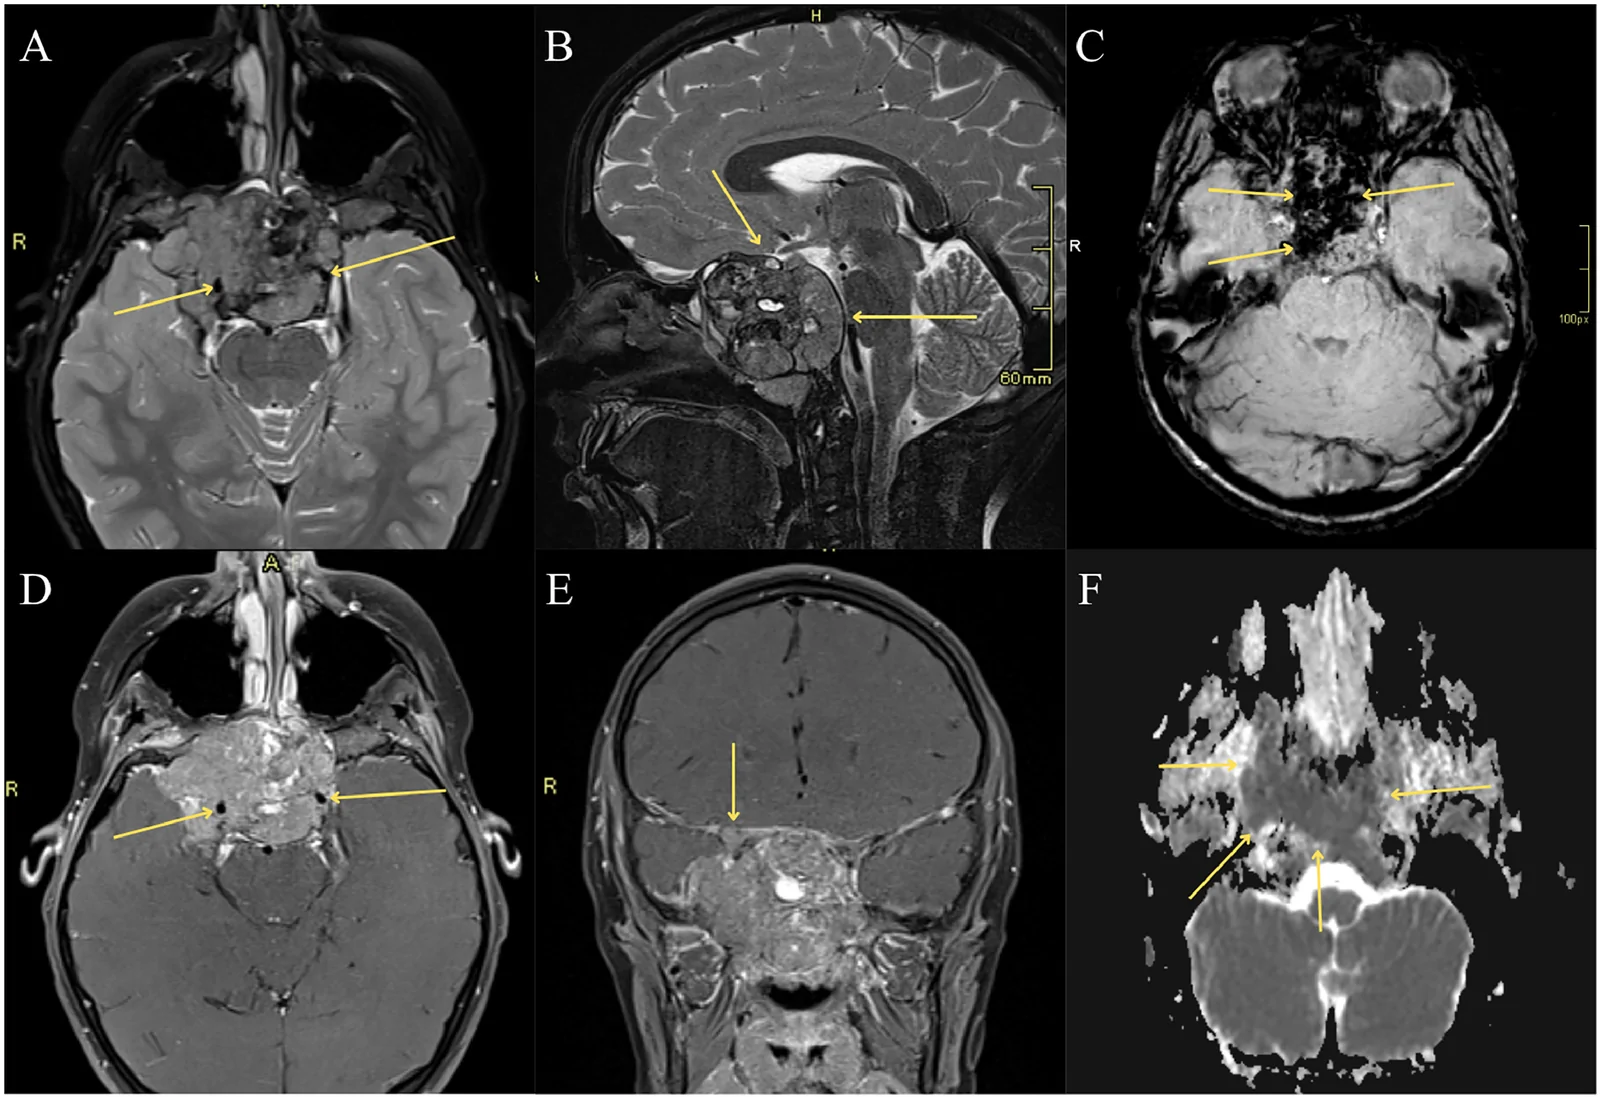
Multiplanar MRI of SMARCB1-deficient sinonasal carcinoma in an 8-year-old patient. (A) Axial T2-weighted image demonstrates a large, heterogeneous central skull base mass, eccentric to the right, with involvement of the sphenoid sinuses and encasement of the bilateral internal carotid artery flow voids (arrows). (B) Sagittal T2-weighted image demonstrates the extent of craniocaudal involvement of the central skull base with extension into the sellar and clival regions (arrows). (C) Axial susceptibility-weighted imaging (SWI) demonstrates multiple foci of susceptibility hypointensity within the lesion (arrows), corresponding to calcification and intralesional hemorrhage. (D) Axial contrast-enhanced T1-weighted image demonstrates heterogeneous enhancement with encasement of the bilateral internal carotid arteries (arrows) and extension toward the right pterygopalatine region. (E) Coronal contrast-enhanced T1-weighted image demonstrates the large heterogeneously enhancing central skull base mass with involvement of the right orbital apex (arrow). (F) Apparent diffusion coefficient (ADC) map demonstrates low ADC values within the lesion (arrows) with a mean value of 0.603 × 10⁻³ mm²/s, consistent with restricted diffusion in a hypercellular neoplasm.
